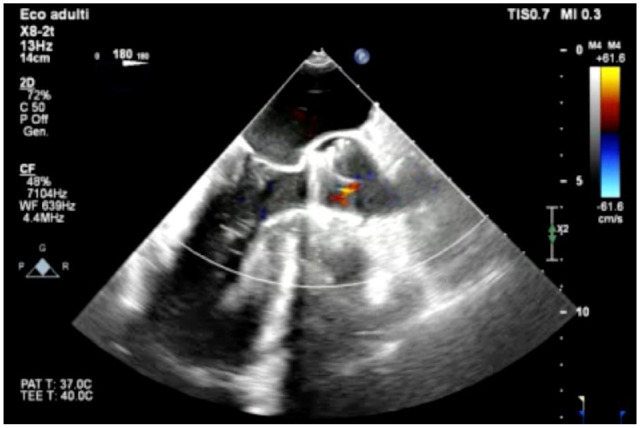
Post–surgical aortic valve replacement transesophageal echocardiography showing absence of intravalvular and paravalvular regurgitation.
